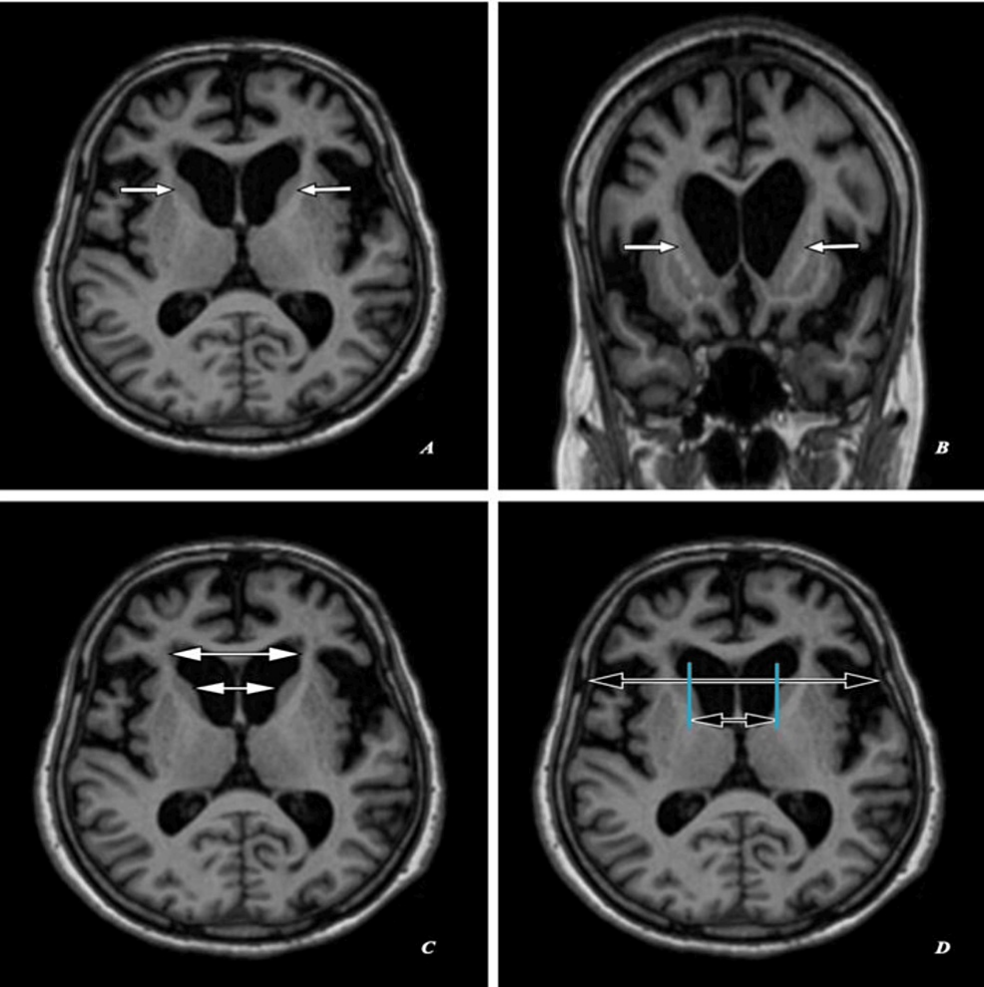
Magnetic resonance imaging (MRI) brain (T1-weighted): (A,C,D) Axial sections and (B) coronal section of the same patient. (A,B) Shows head of bilateral caudate nuclei mild atrophy with ex-vacuo mild dilatation of frontal horns of lateral ventricles (short white arrows). (C) Shows frontal horn width (large double white arrow) to intercaudate distance (small double white arrow) ratio (FH/CC) of 1.6, mildly decreased. (D) Shows an intercaudate distance (small double black arrow) to inner table width (large double black arrow) ratio (CC/IT) of 0.24, mildly increased.
